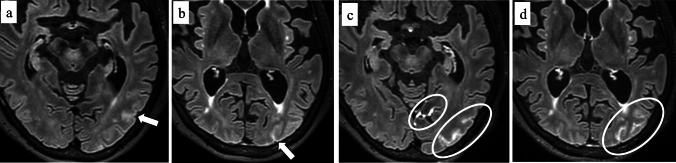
A 72-year-old female with amyloid-related imaging abnormalities-effusion (ARIA-E), eight weeks after receiving lecanemab for the treatment of mild cognitive impairment. There are no symptoms associated with ARIA-E. Non-contrast-3D FLAIR (a, b) shows hyperintensities in the sulci of the left posterior lobe (arrows) without new hemorrhagic lesions. Contrast-enhanced 3D FLAIR (c, d) shows enhancement in the sulci of the left posterior lobe (circles)
